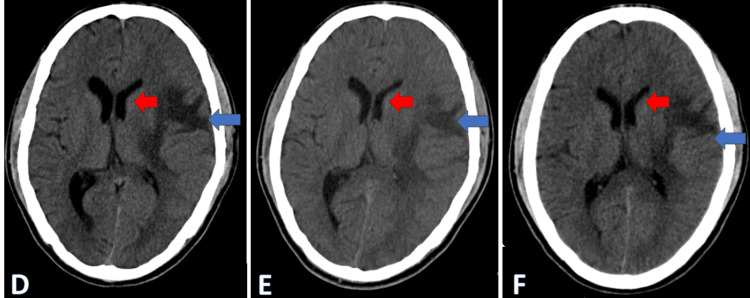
Serial CT brains (axial view) done on Day 13 (D), Day 21 (E), and Day 28 (F) showing a decrease in mass effect on the left ventricle (red arrow) and cerebral edema (blue arrow) CT: computed tomography
